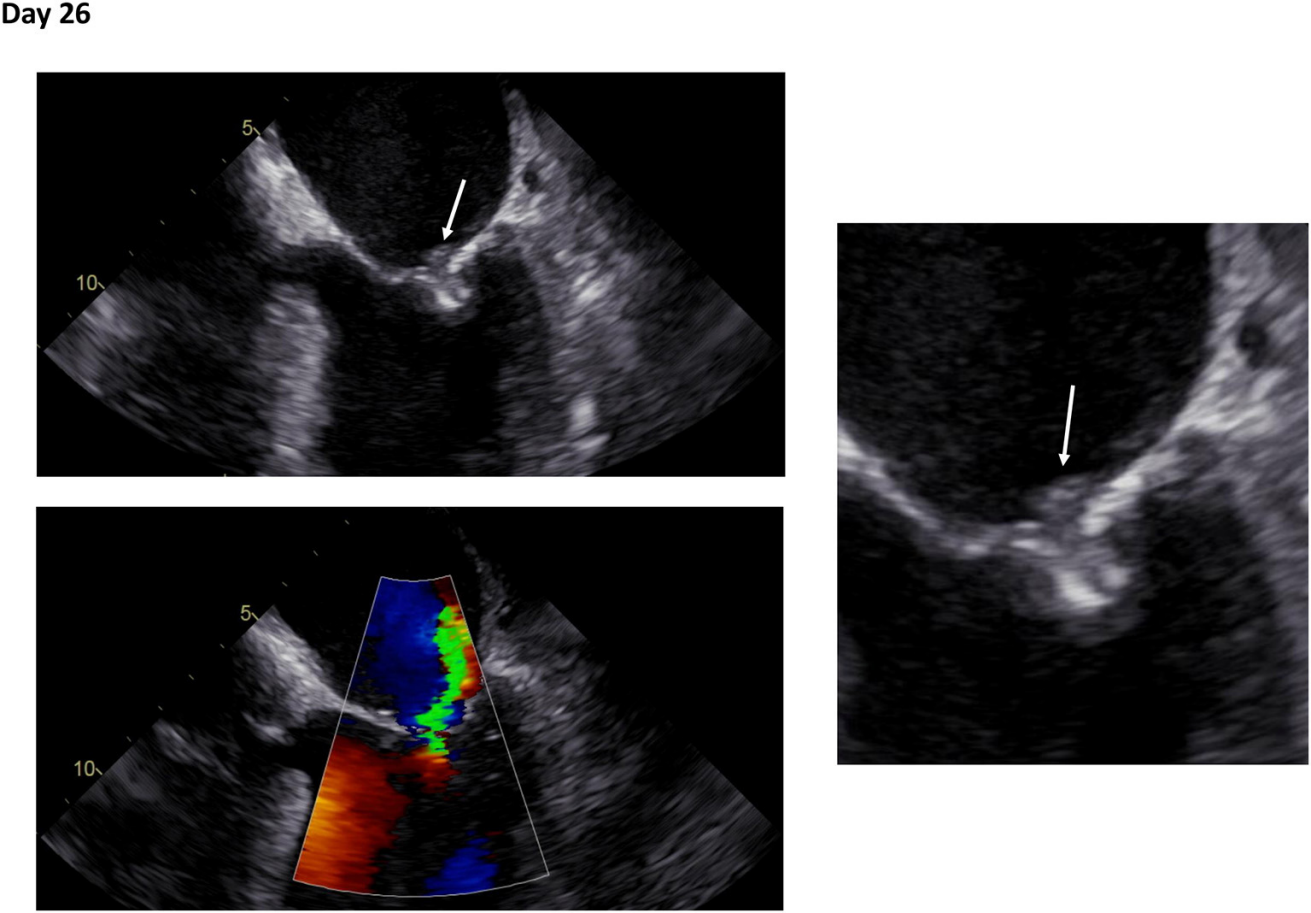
Initial transesophageal echocardiography in recurrent fever and persisting bacteremia. Transesophageal echocardiography at day 26 following mitral valve edge-to-edge repair. Right: Zoom on mitral valve. White arrows indicate an echogenic structure suspicious for vegetation (Day 26: Supplementary Videos 1, 2).
